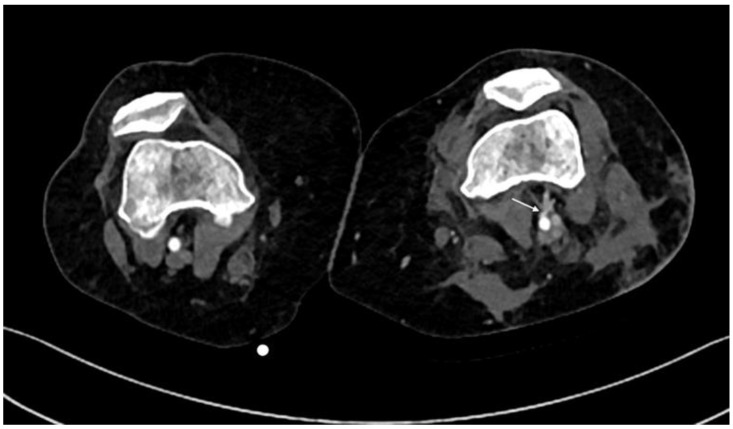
CTA, axial planes. Left popliteal arteriovenous fistula: A direct connection between the artery and the vein with early venous enhancement in the arterial phase and communicating channel with the artery can be detected (arrow).
